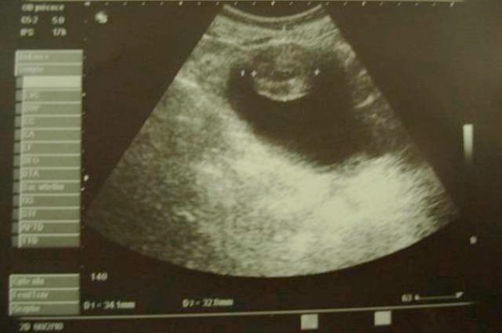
Ultrasonography showing a heterogeneous mass located in the bladder dome.
